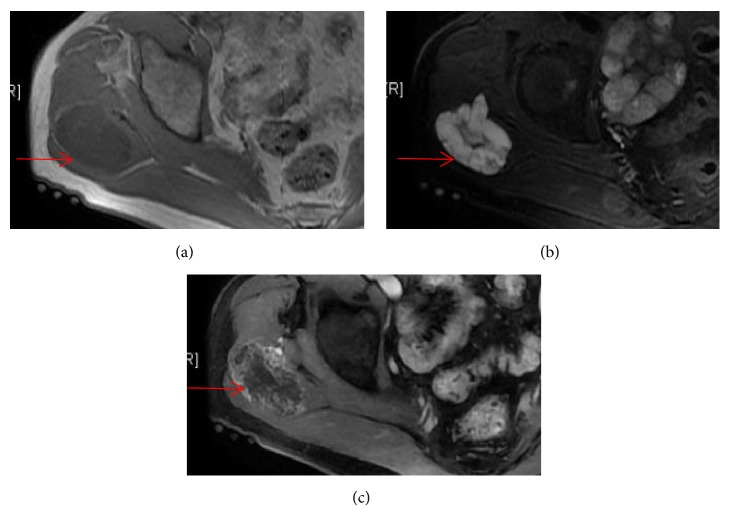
Images obtained from a 52-year-old man with EMC of the right gluteus. (a-b) T_1_WI and T_2_WI: the lesion (red arrow) demonstrated lobulated mass with hyposignal intensity on T1-weighted sequences and hypersignal intensity on T2-weighted sequences; it showed enhancing internal septation after injection of gadolinium (c).
